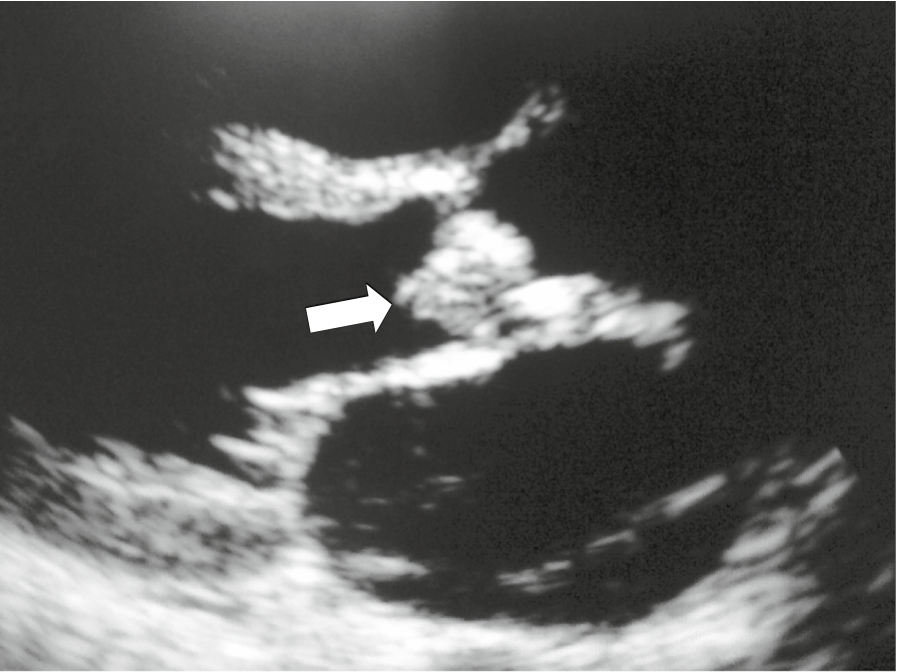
Transthoracic echocardiography showing vegetation (white arrow) on the aortic valve
